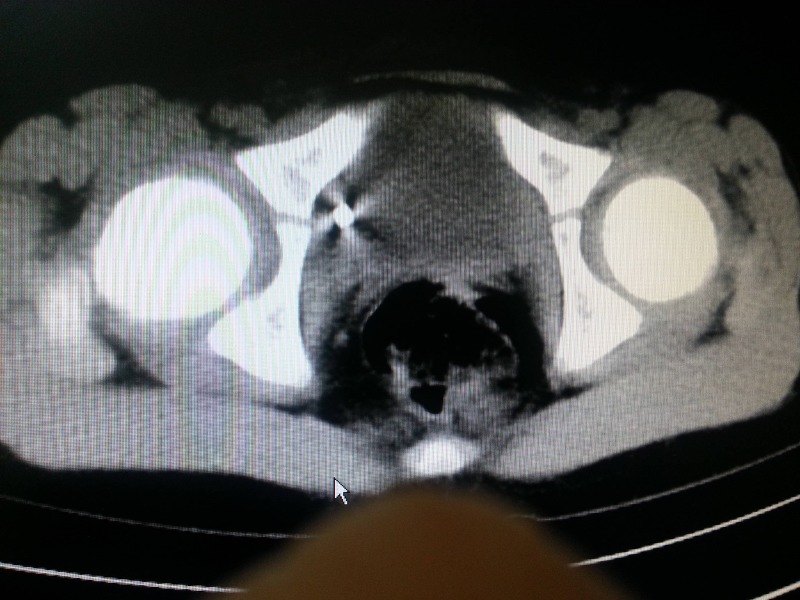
Figure 1:CT scan showing bullet in the pelvis near the right side of the urinary bladder.
